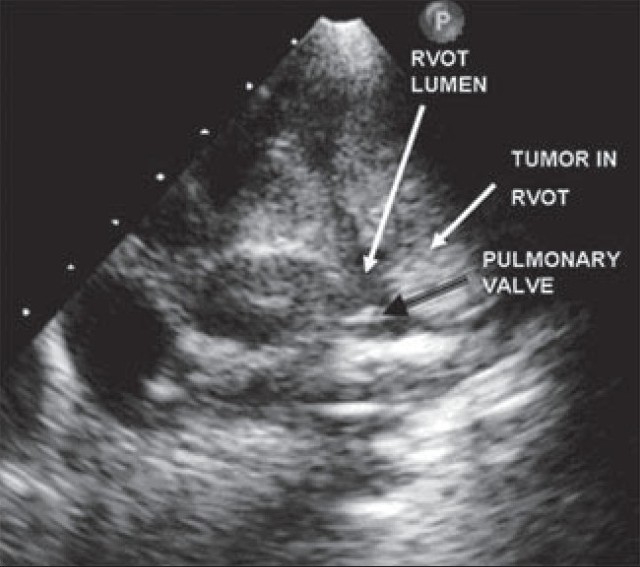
Extension of the tumor into the RVOT showing the tumor filling the right ventricle in parasternal short axis view; RVOT, right ventricular outflow tract
